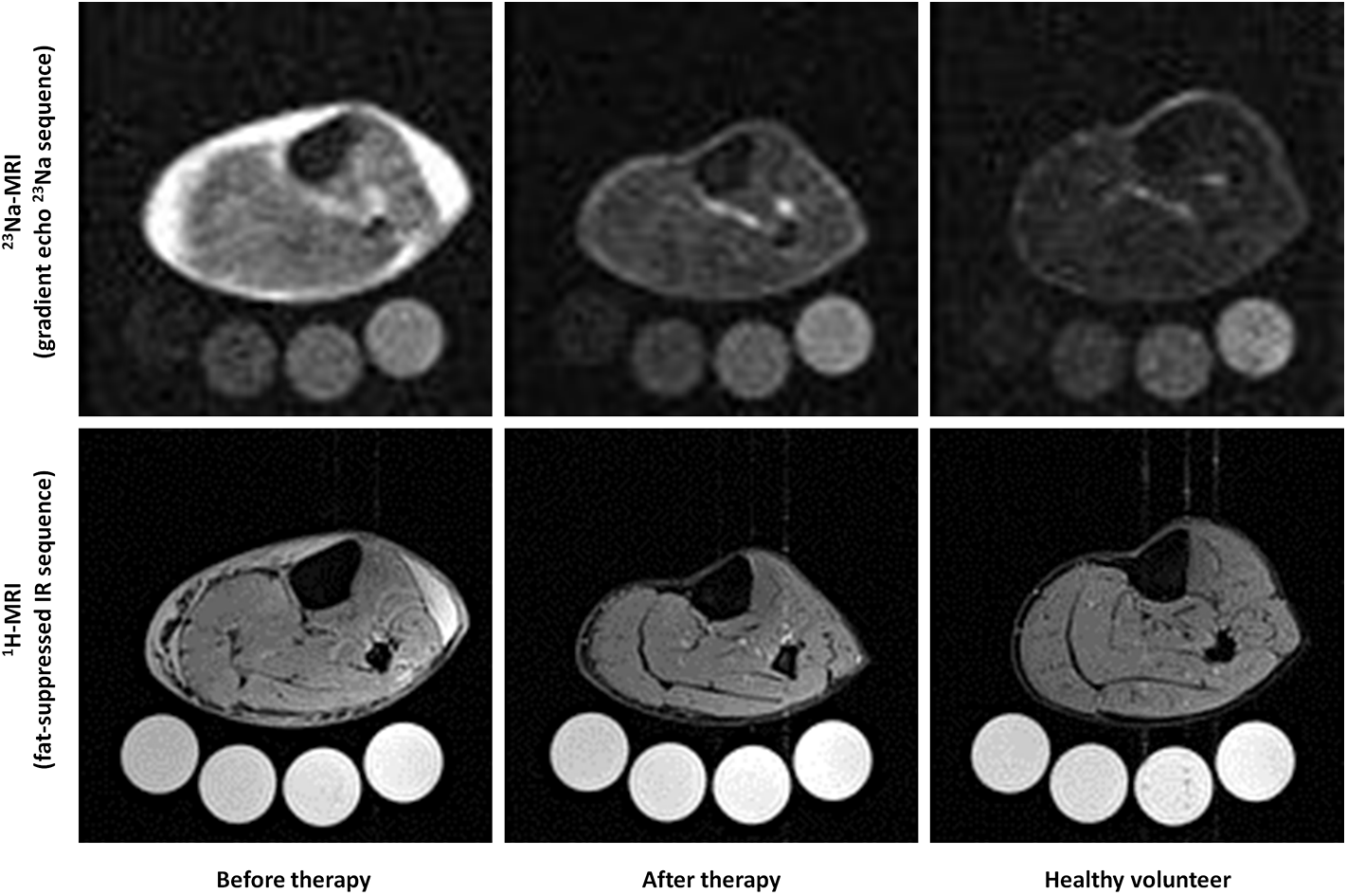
Exemplary ^23^Na/^1^H-MRI-images of a patient with acute heart failure before and after diuretic treatment and of a matched healthy subject. Upper row:
